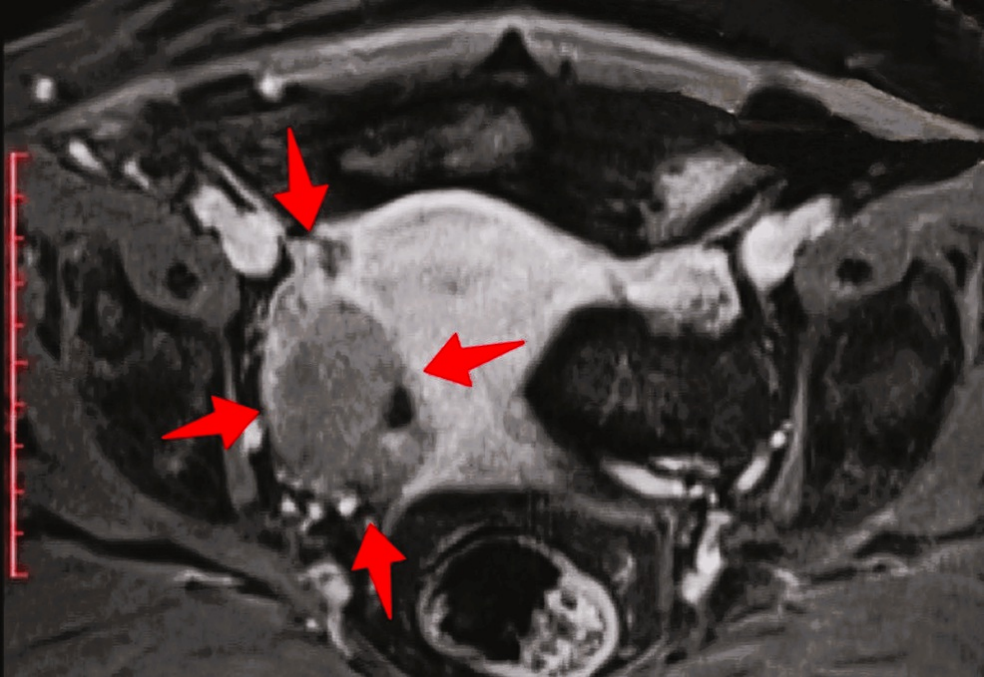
MRI of ovarian fibroma (our case) In the anatomical position of the right adnexa (red arrows) the presence of a pathologic space-occupying lesion that occupies the ipsilateral ovary is highlighted, without the presence of imaging of normal ovarian tissue
